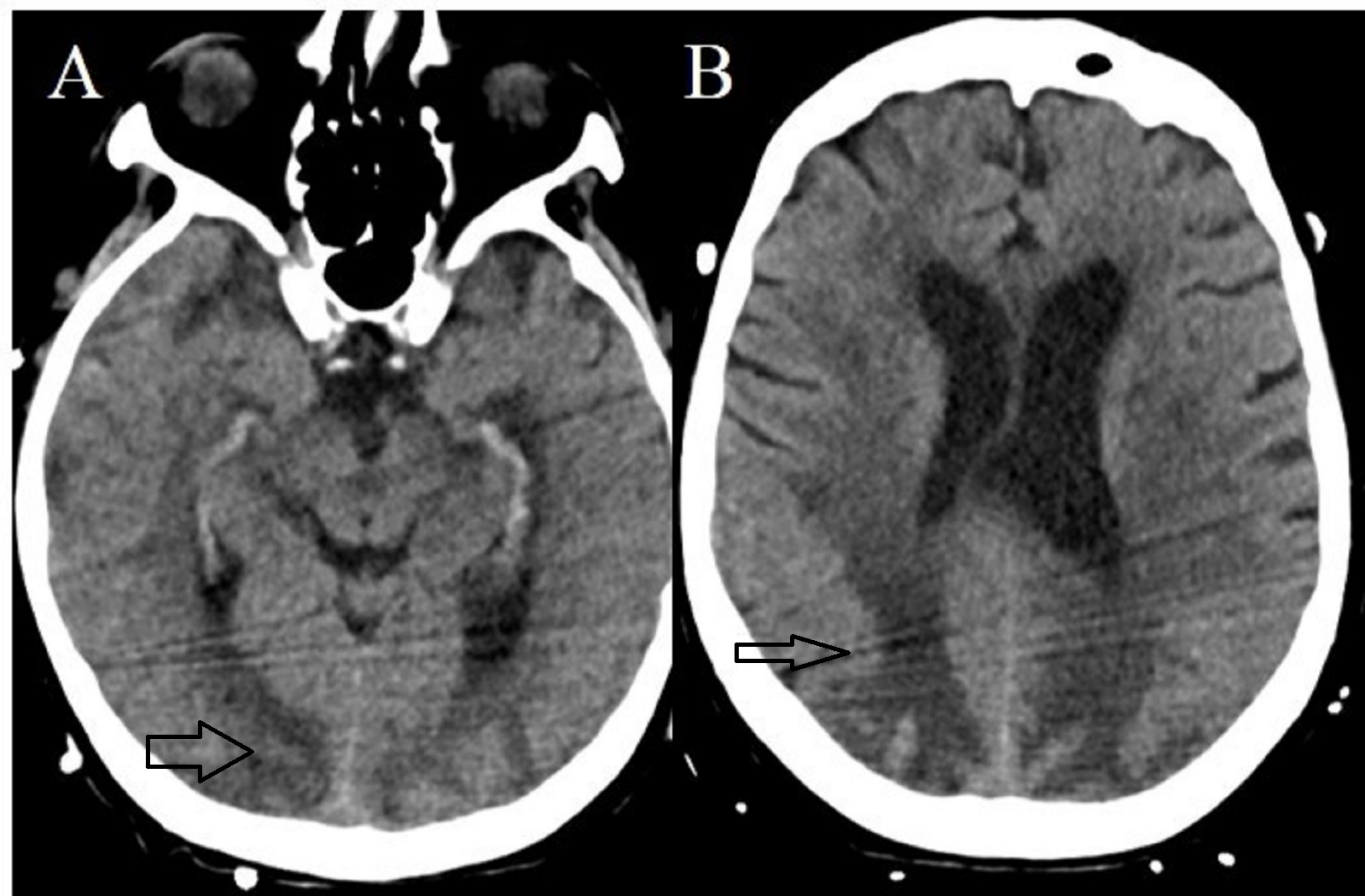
Computed tomography (CT) of the head without contrast There is hyperdensity within the bilateral posterior quadrants consistent with hemorrhagic transformation.
